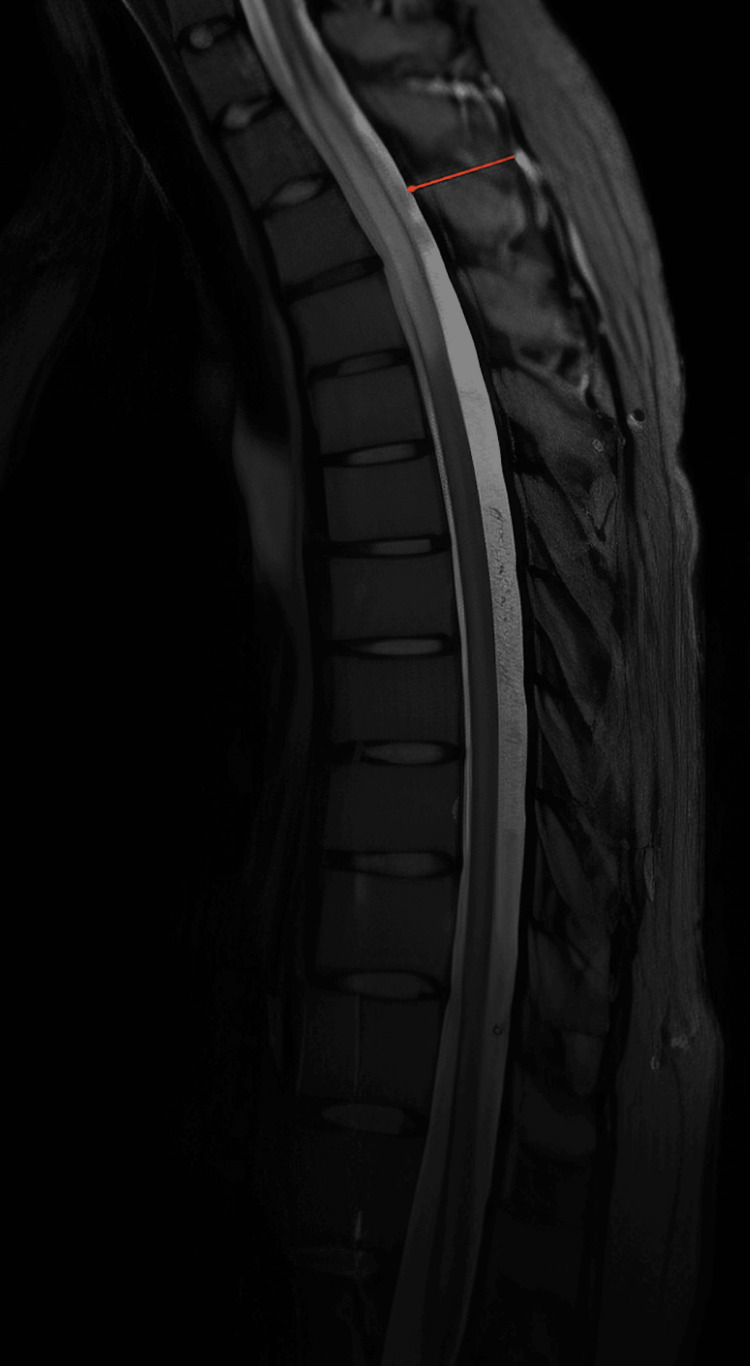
The patient's thoracic spine magnetic resonance imaging showing focal long segment area of abnormal hyperintensity in the thoracic spinal cord with cord swelling at the T1 through T5 levels with enhancement (red arrow).
